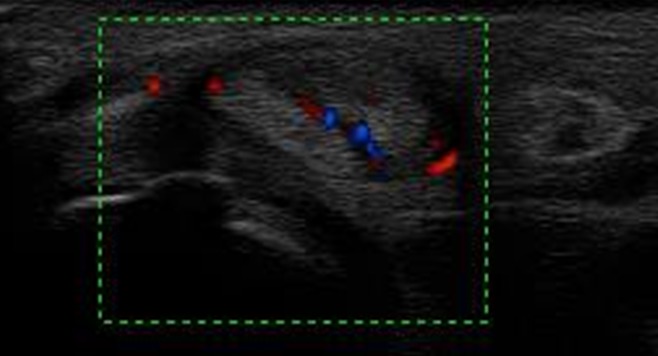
Coupe transversale du tendon fléchisseur du 2ème rayon droit: épanchement liquidien dans la gaine tendineuse des tendons fléchisseurs superficiel et profond du 2ème rayon droit, hyper vascularisée au doppler puissance
